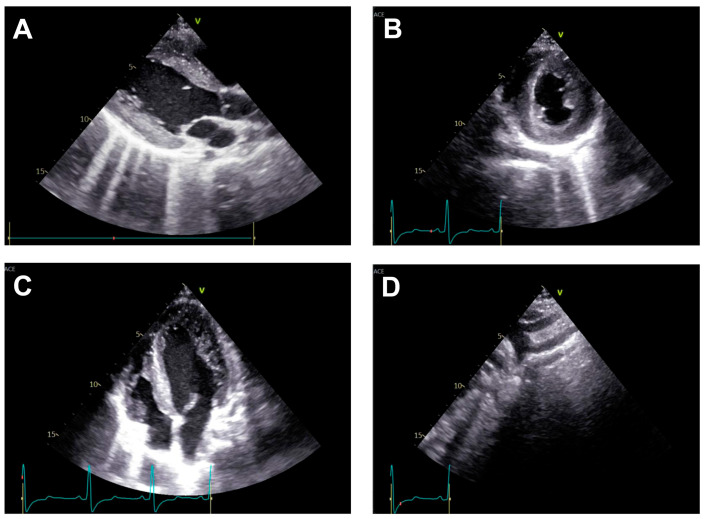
Transthoracic echocardiographic aspect. (A). Parasternal long-axis view at the level of the left ventricle (LV)—significant left ventricular hypertrophy (LVH) with normal morphology of the aortic valve (AV) and mitral valve (MV). (B). Parasternal short-axis view at the level of the papillary muscles, confirming left ventricular hypertrophy (LVH) without evidence of pericardial effusion. (C). Apical four-chamber view further highlighting pronounced left ventricular hypertrophy (LVH). (D). Suprasternal window showing the emergence of the left common carotid artery and left subclavian artery, followed by abrupt narrowing and total occlusion of the descending thoracic aorta.
